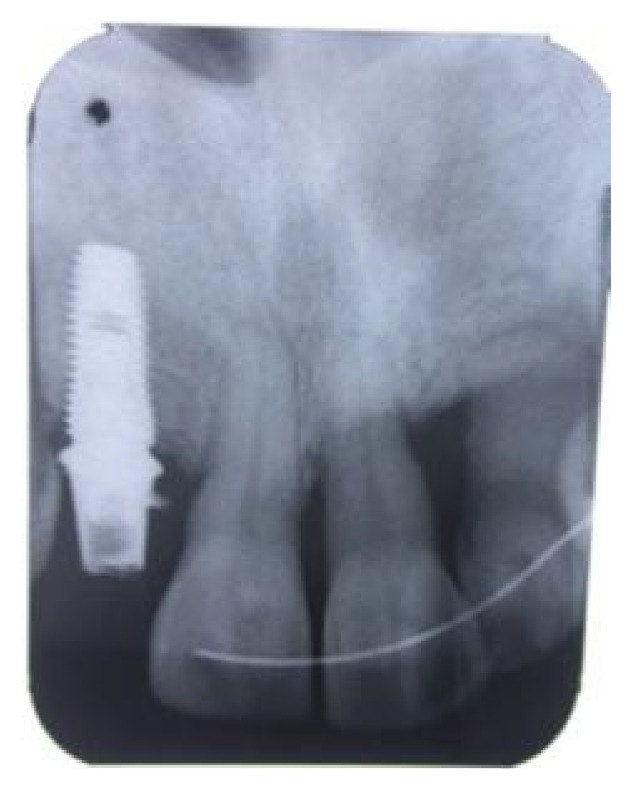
A radiograph showing the implant placement.
